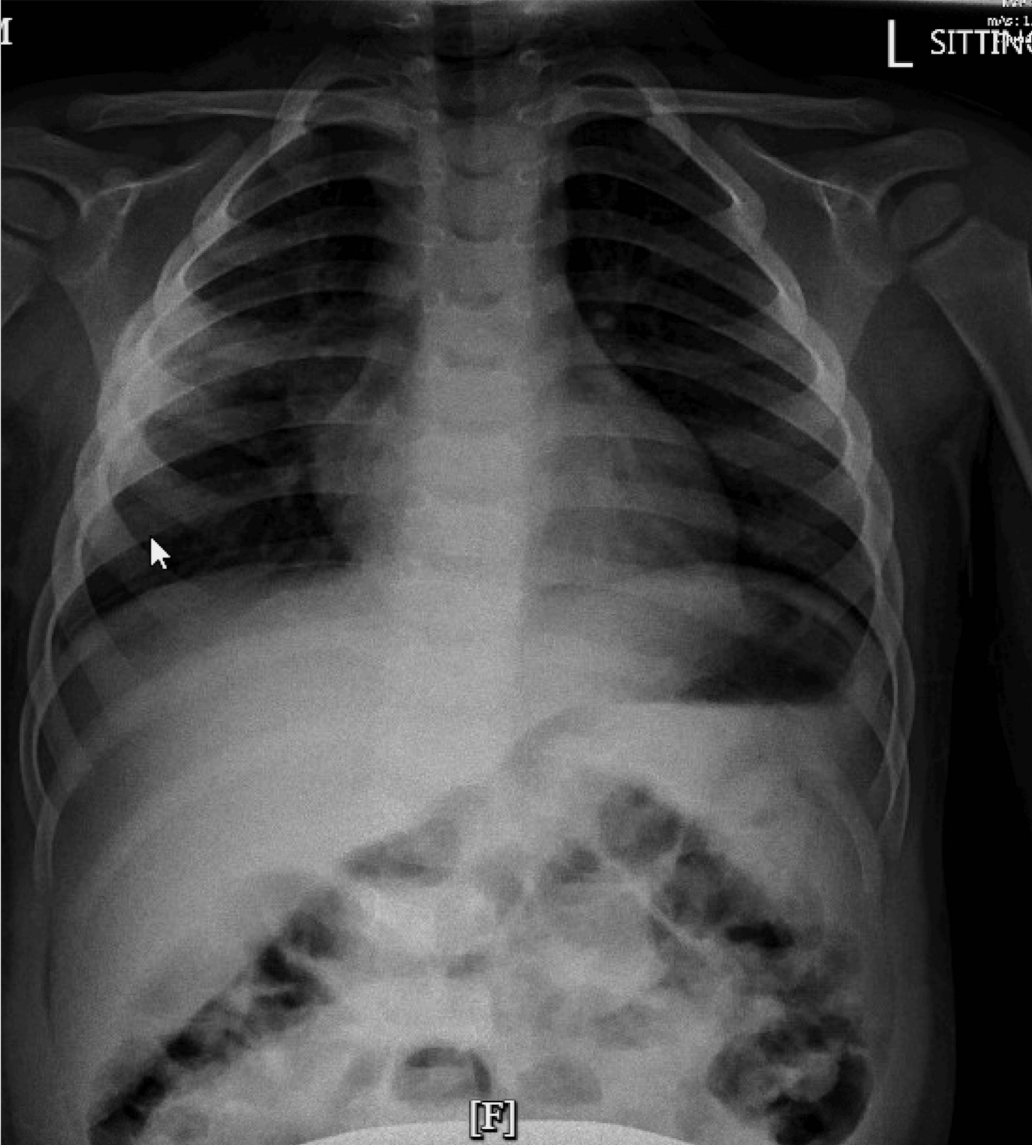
Chest X-ray, three months post admission; right pleural effusion and hazy opacities along the lateral aspect of the right lung, with decreased opacity since prior examination.
